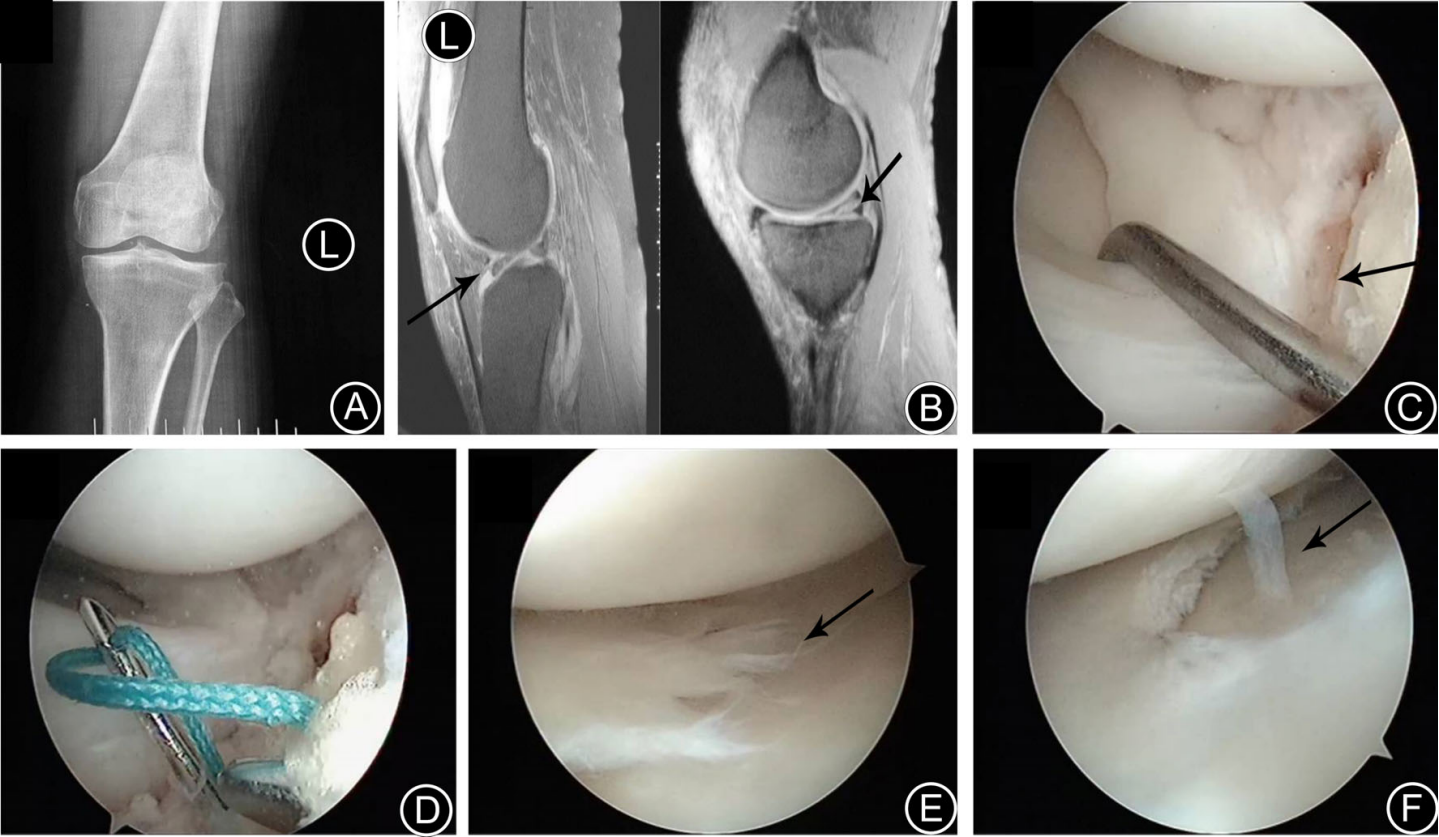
A 53‐year‐old female patient with combined injury of posterior horn medial meniscus and anterior horn lateral meniscus in the left knee. (A) Anteroposterior X‐ray film before operation; (B) Sagittal MRI before operation; black arrow indicates longitudinal tears of anterior horn lateral meniscus and horizontal tears of posterior horn medial meniscus; (C) Longitudinal tears of anterior horn lateral meniscus under arthroscope (arrow); (D) The anterior horn lateral meniscus after suturing; (E) Horizontal tears of posterior horn medial meniscus under arthroscope (arrow); (F) The posterior horn medial meniscus after menisectomy.
